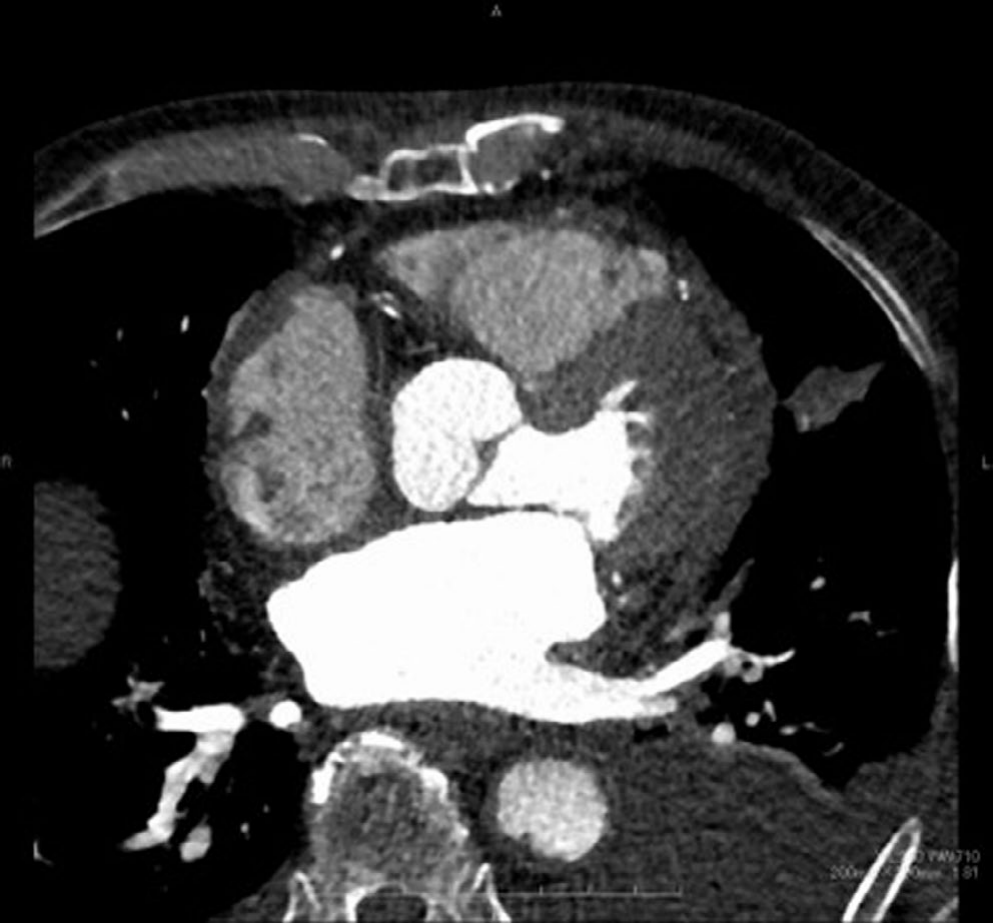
Follow‐up computed tomography showing no mass in the left atrium
